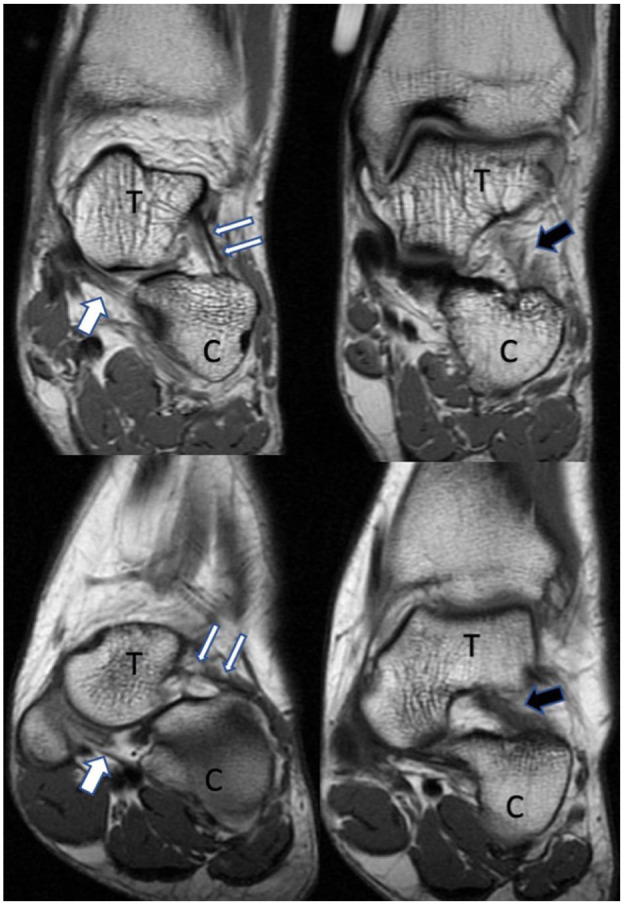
Coronal T1-wighted magnetic resonance images in a control patient (top images) and a PCFD patient (bottom images). Images highlight the cervical ligament (thin white arrows), spring ligament (thick white arrow), and interosseous talocalcaneal ligament (thick black arrow). Note increased horizontal orientation of the cervical and interosseous talocalcaneal ligaments in the PFCD patient compared with the control patient. C, calcaneus; PCFD, progressive collapsing foot deformity; T, talus.
